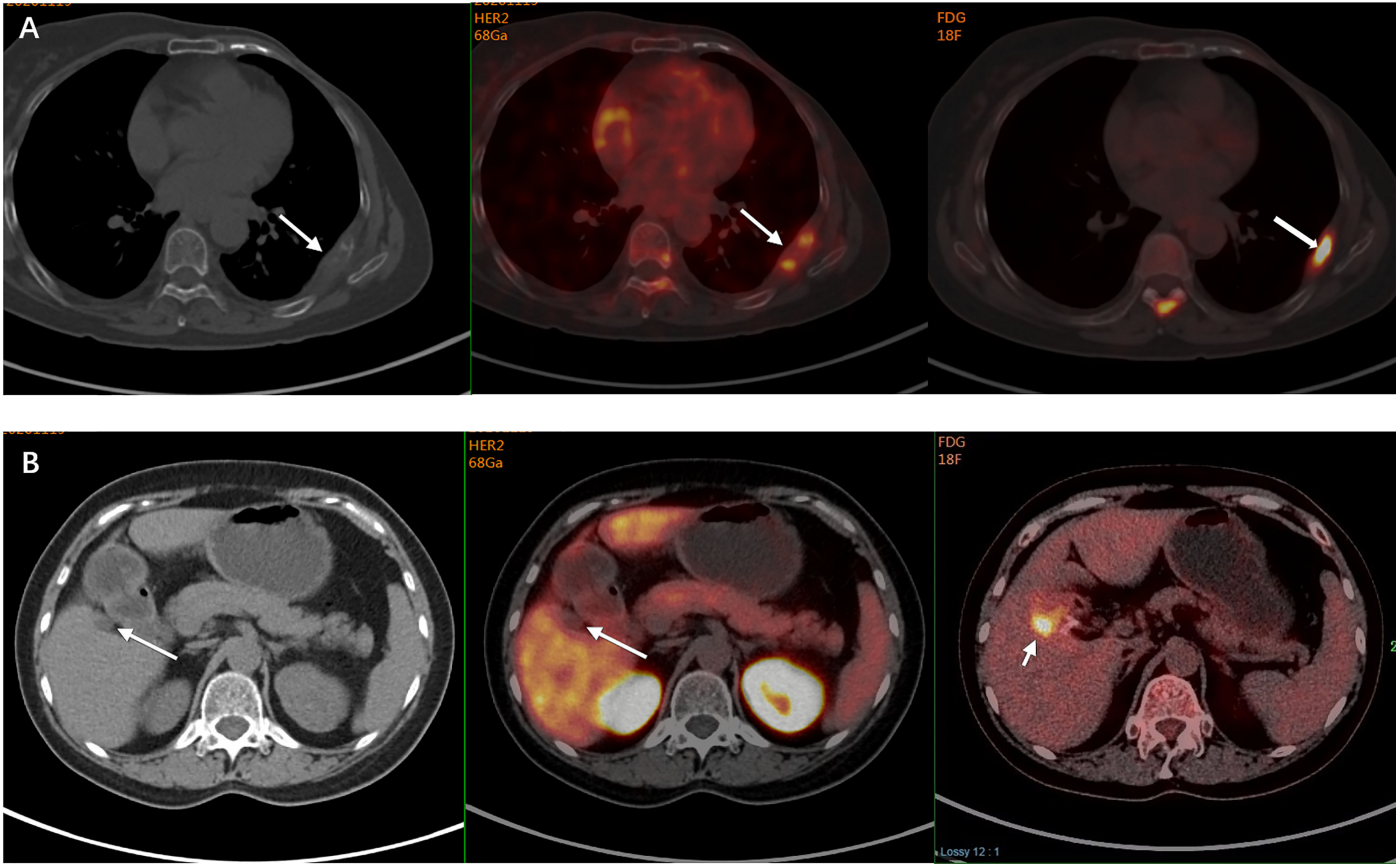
A 60-year-old woman was diagnosed with primary ER-negative/HER2-positive invasive ductal breast carcinoma. Two years later, she developed bone metastases in her left posterior ribs. PET/CT also demonstrated a hypodense shadow in the right anterior lobe of the liver with increased FDG uptake on the FDG PET, and a liver metastasis was suspected. Subsequent ^68^Ga-HER2 affibody PET/CT showed that the HER2 uptake in the left posterior rib was increased (arrow, SUV_max_ of 4.4) (A), while no ^68^Ga-HER2 affibody avidity was found in the right anterior lobe of her liver close to the gallbladder fossa though increased FDG uptake was shown in the same site (arrow) (B). The pathology of liver biopsy revealed that the suspicious lesion in her liver was actually the infiltration of inflammatory cells in the liver portal area. The manifestation on ^68^Ga-HER2 affibody PET/CT scan was consistent with the pathology, which suggested the extended use of HER2 PET in the discrimination of metastases.
